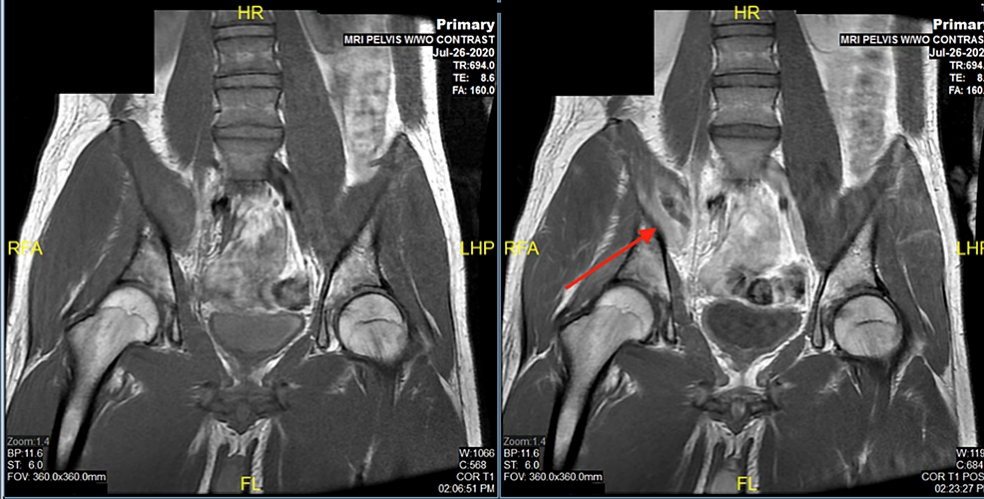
MRI of the pelvis: septic right sacroiliac joint with decompression of pus from the joint resulting in secondary pyomyositis
